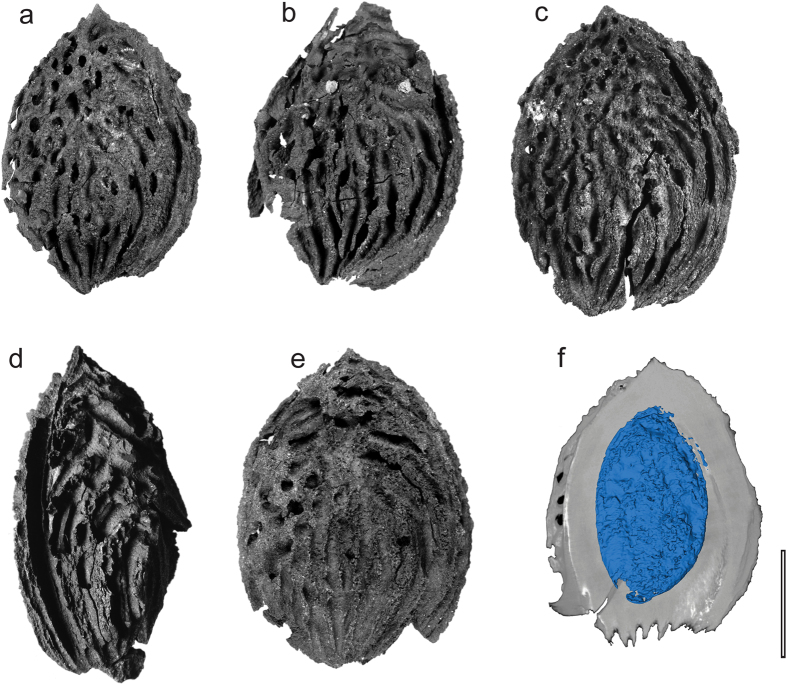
Prunus kunmingensis*. (a–e) KUN PC2015001-KUN PC2015005. (f) CT scan showing longitudinal section and seed (KUN PC2015001). Scale bar = 1 cm. See the three dimensional reconstruction in Animation S1.
